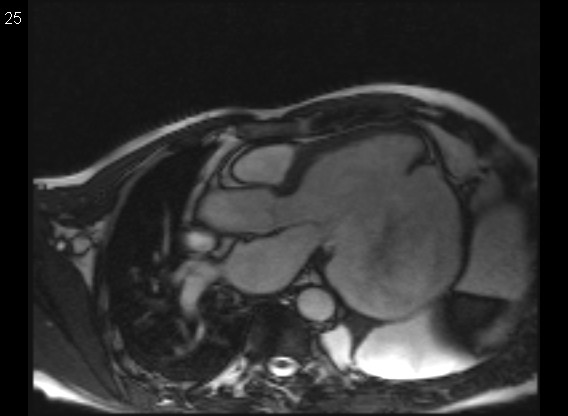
Preoperative magnetic resonance imaging. Left ventricular outflow tract. Giant left ventricular aneurysm of the lateral wall, strictly confined to the submitral region between anterolateral and posteromedial papillary muscle. Narrow aneurysma neck stretching in length from the apex up to 1 cm below the mitral valve annulus with the aneurysmal sac bulging to the thoracic wall. Signal alteration as sign of blood stasis in left ventricle. Spontaneous contrast on echocardiography. Of note: thin aneurysmal sac.
